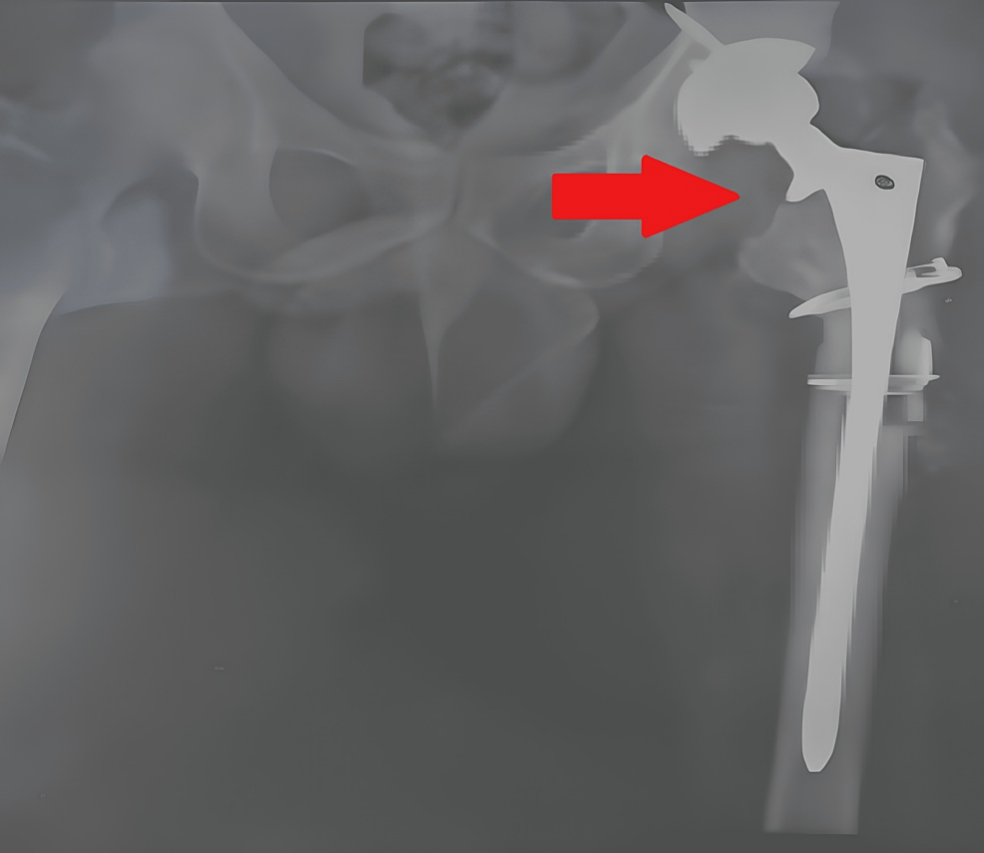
Postoperative X-ray after final metal implant surgery (September 27, 2022)
